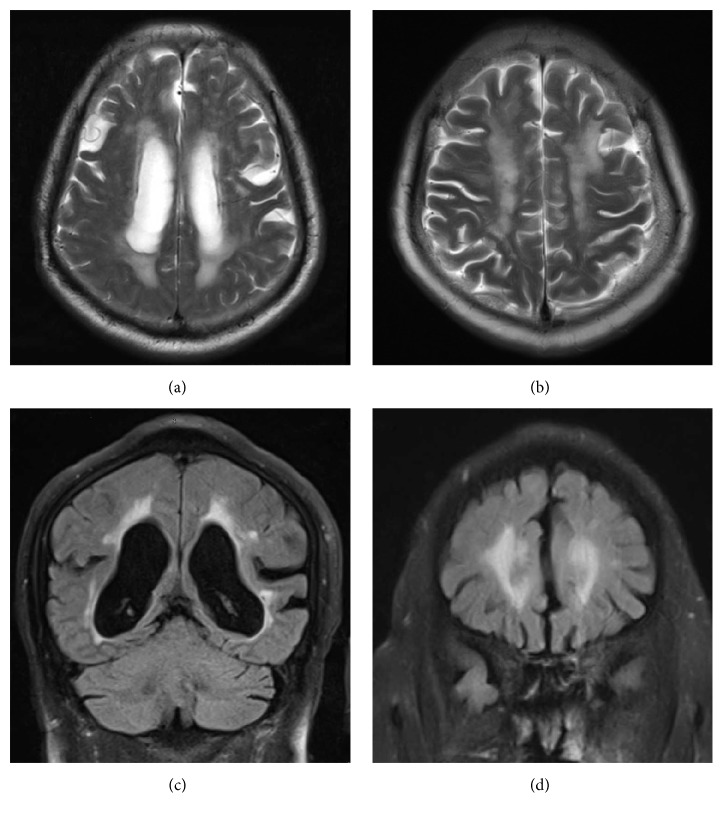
Neuroimaging features of white matter lesions: (a) periventricular white matter lesions on T2-weighted MRI. (b) Deep/subcortical white matter lesions on T2-weighted MRI. (c) Periventricular white matter lesions on MRI (FLAIR image). (d) Deep/subcortical white matter lesions on MRI (FLAIR image). FLAIR: fluid-attenuated inversion recovery.
